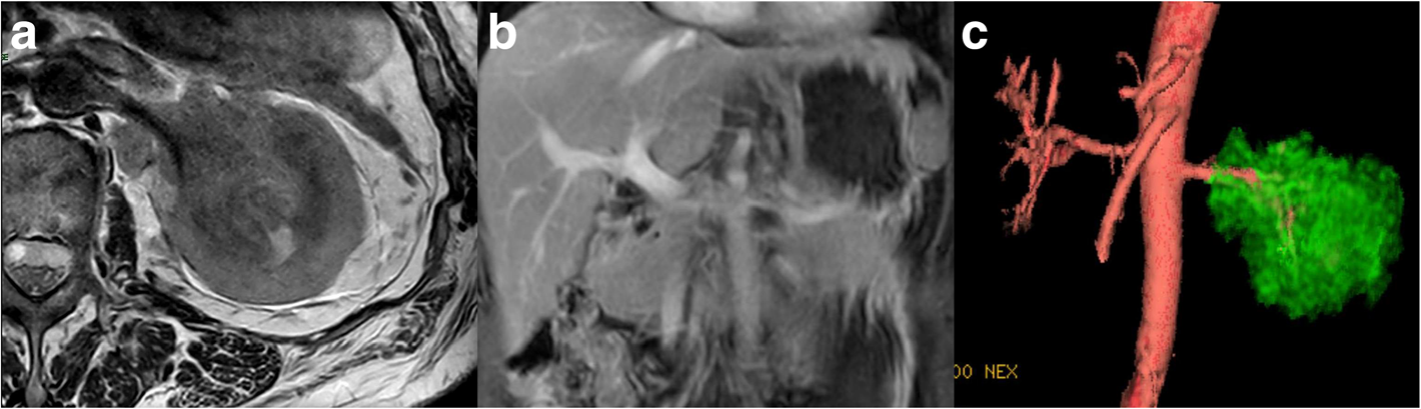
Radiological findings of renal pelvic TCC with renal vein thrombosis. a Case 3: axial MRI image showed a soft tissue mass in the region of the left renal sinus with parenchyma and perirenal fat infiltrated. The signal of soft tissue was also seen in the left renal vein. b Case 3: coronal MRI image showed renal vein thrombosis. c Case 3: MRI 3D reconstruction image showed a mass in the region of the left kidney
